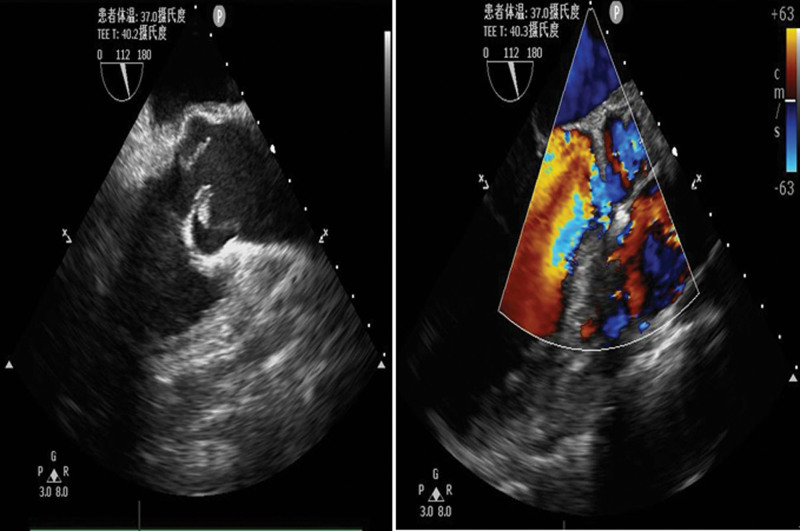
TEE showed significant aortic regurgitation and vegetation. TEE = transesophageal echocardiography.
